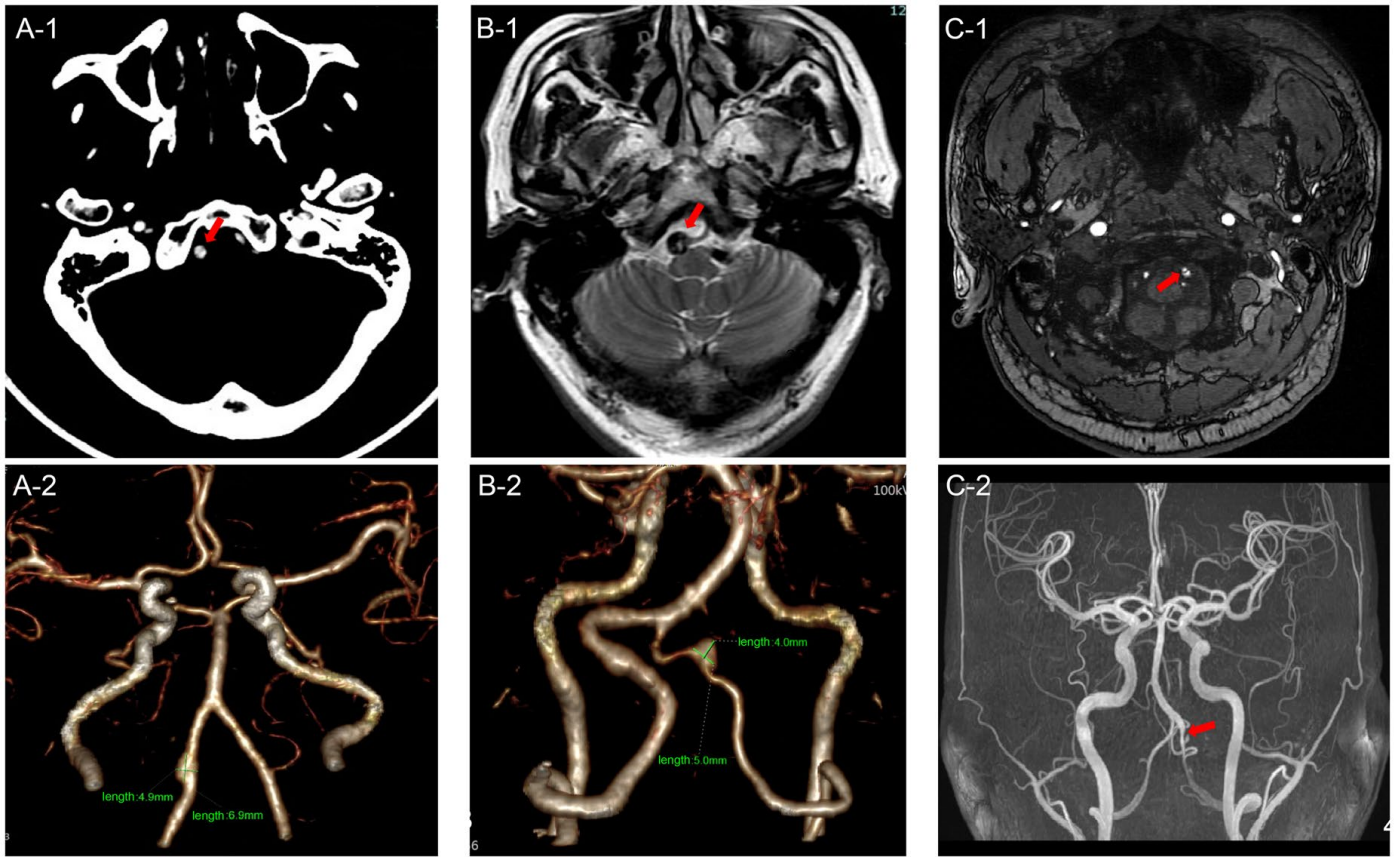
The imaging features of spontaneous intracranial artery dissection. A. CTA angiography of the head and neck in a 62-year-old male patient. (A-1) CTA raw thin-layer image showing a linear irregular isodense intima-media flap shadow visible in the lumen of the right vertebral artery (arrowed). (A-2) CTA volume reconstruction showed local tumor-like expansion of the right vertebral artery, with a size of about 7*5mm. B. A 54-year-old male patient with a diagnosis of limited dissection of the V4 segment of the right vertebral artery. (B-1) Magnetic resonance diffusion weighted imaging of the head showed high signal intima flap of the right vertebral artery (arrowed). (B-2) CTA volume reconstruction showed local nodular protrusions in the right vertebral artery V4 segment, with a size of about 4*5mm. C. High-resolution magnetic resonance enhancement imaging of the cervical and intracranial arterial vessel walls in a 49-year-old male patient. (C-1) Low and short T1 signal double-lumen shadows were seen in the proximal segment of the left vertebral artery, and the local true lumen was severely narrowed(arrowed). (C-2) 3D time-of-flight MRA (TOF⁃MRA) shows stenosis with dilatation of the intracranial segment of the left vertebral artery(arrowed).
